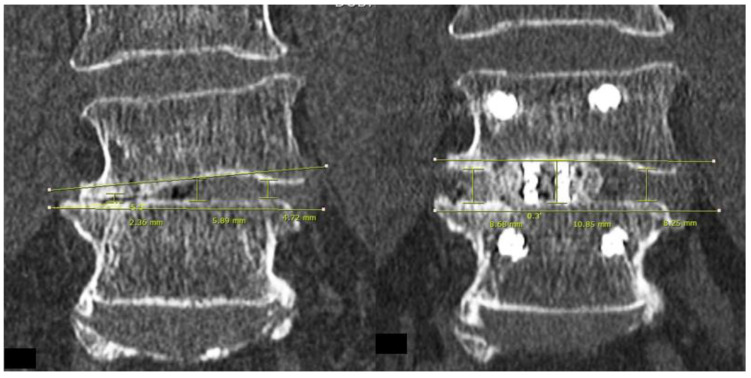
Left picture was pre-operative and right picture was post-operative mid Coronal Cut of CT Lumbar Spine of L4/5, we measured the pre-operative and post-operative mid Coronal Disc height and Coronal Wedge Angle.
